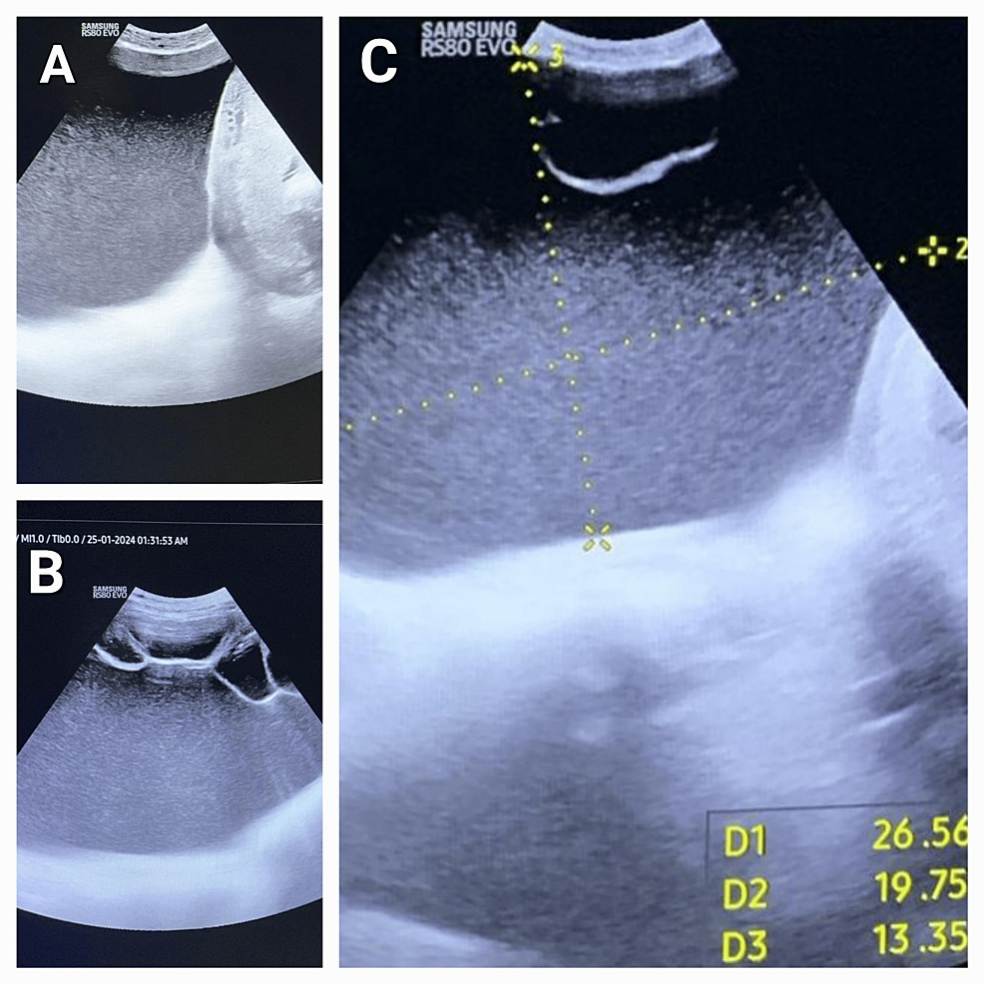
Ultrasonographic image of the ovarian cyst. (A) Ovarian cyst abutting the uterus with the placenta; (B) large multilocular cyst with homogenous low-level echoes in the left adnexal region extending into the abdomen; (C) dimensions of the cysts: 26.56 cm × 19.75 cm × 13.35 cm.
